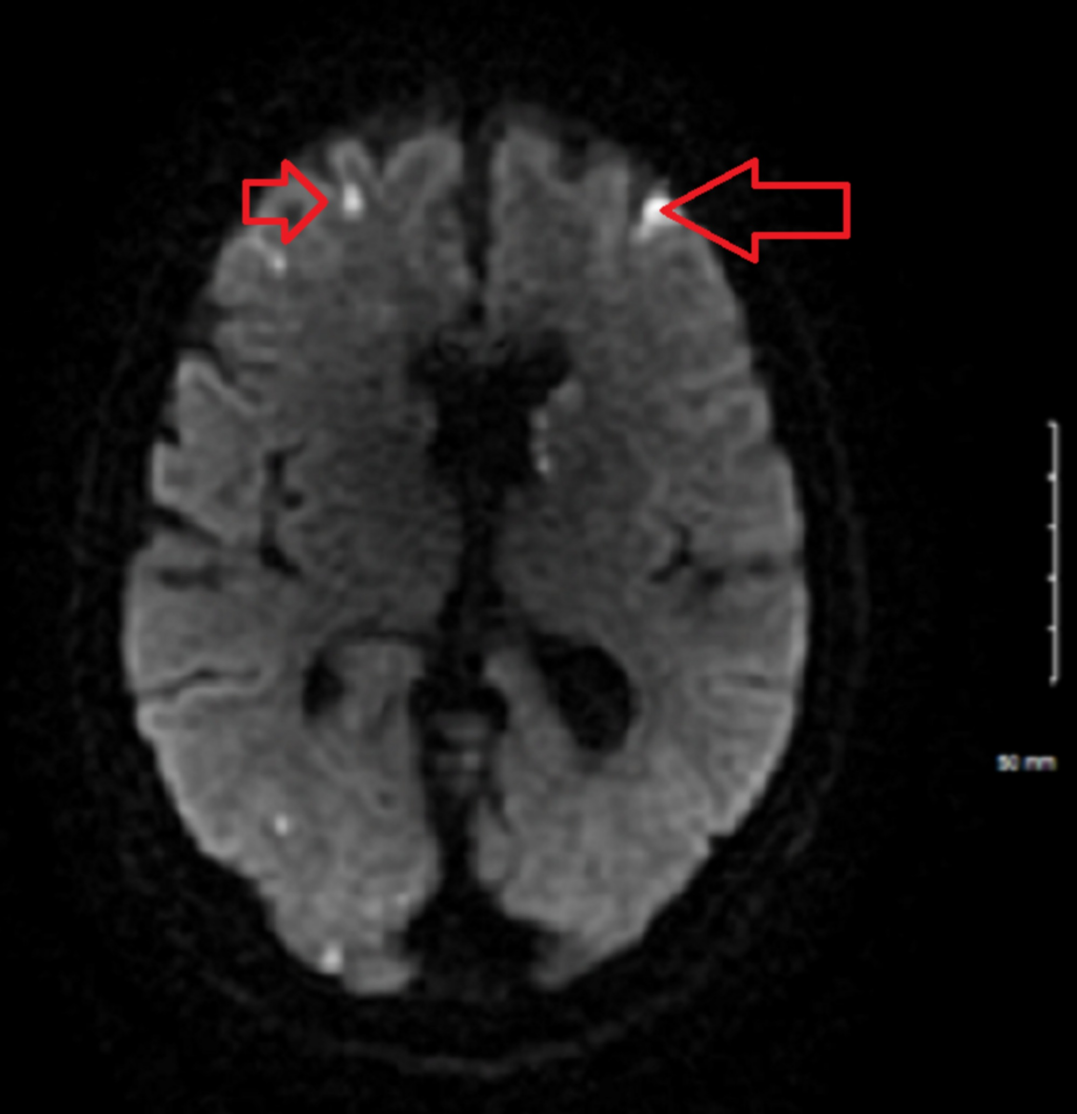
MRI of brain to rule out stroke The arrows indicate the extensive shower emboli found on the MRI.
